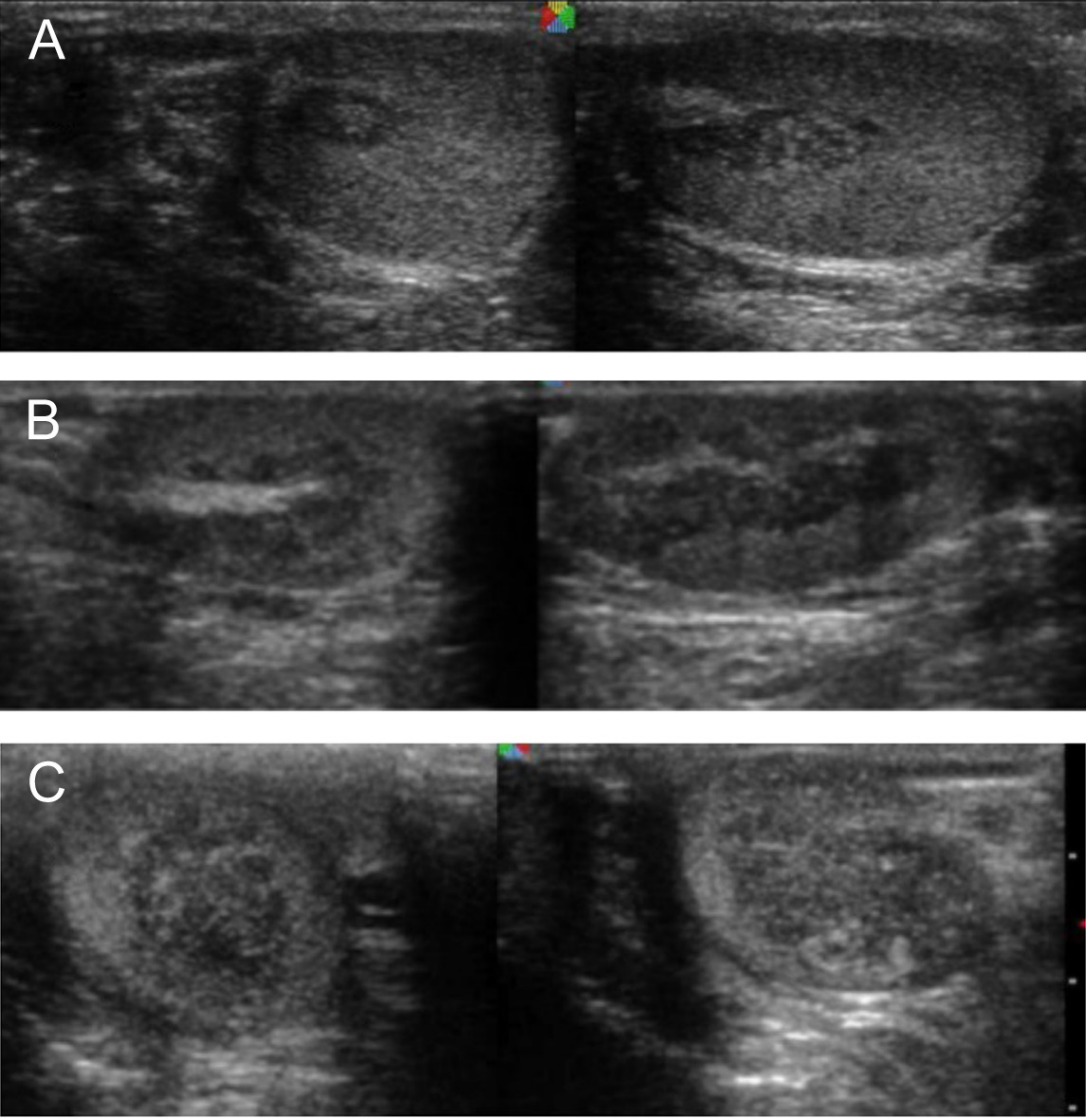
Testicular ultrasonography in patients showing TART location around testicular hilus. (A) Patient 6. (B) Patient 2. (C) Patient 7.
